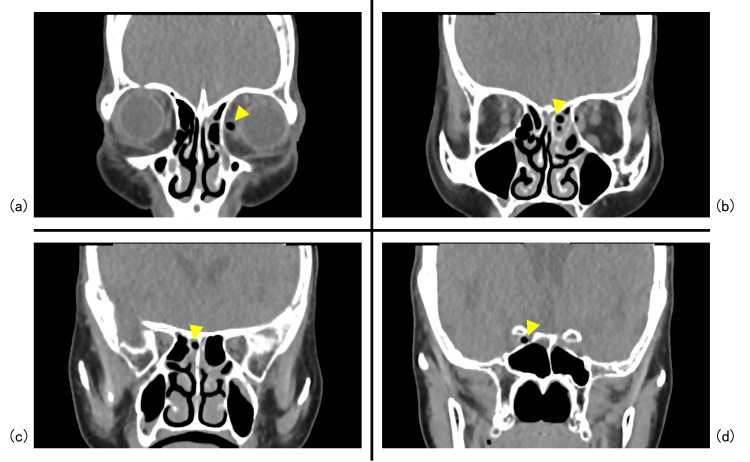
Preoperative coronal CT findings First, in the coronal section, the foreign body (yellow arrowhead) entered from the left medial orbit (a), passed through the left posterior ethmoid sinus (b) and right sphenoid sinus (c), and reached the vicinity of the right internal carotid artery (d).
